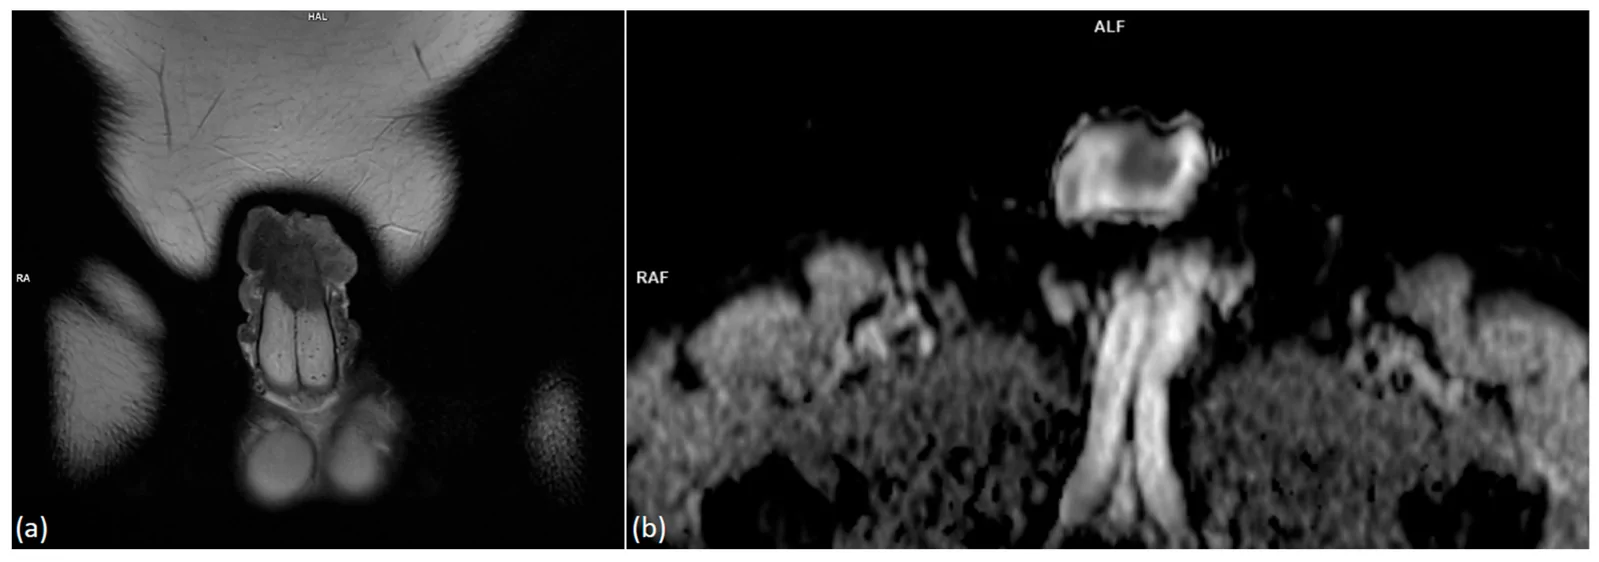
Corpus cavernosum invasion (T3): (a) coronal T2-weighted image; (b) corresponding restricted diffusion on ADC map.
